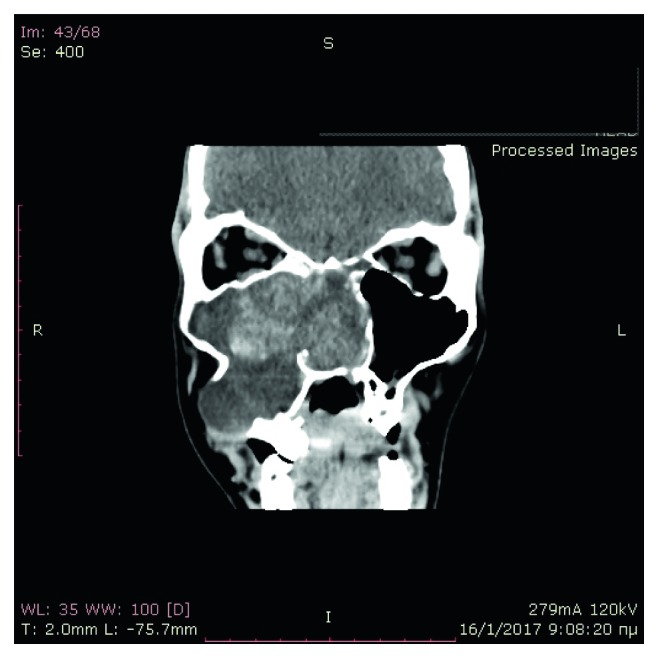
Preoperative CT, coronal plane.
